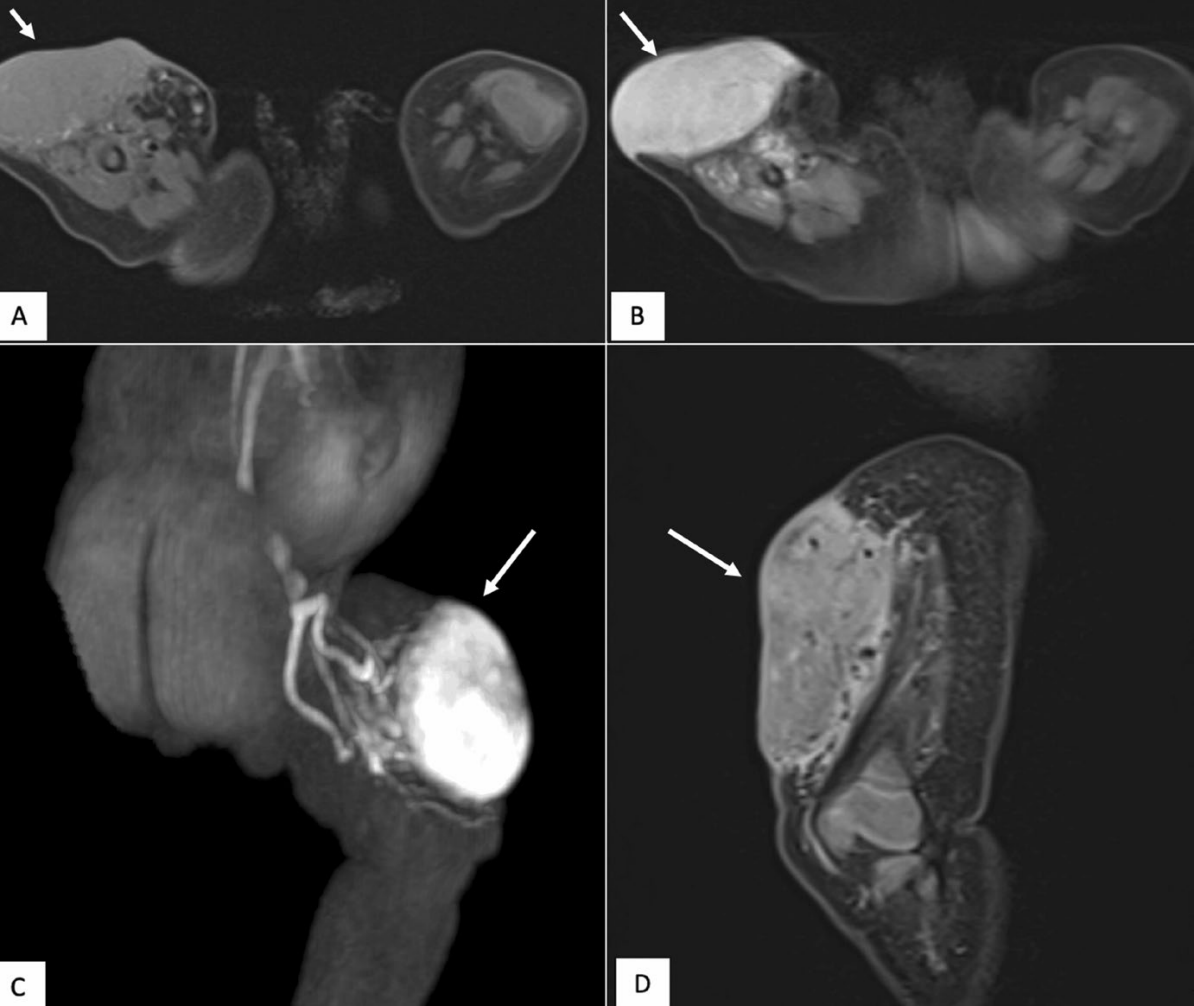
MRI Imaging of Subcutaneous Hemangioma in the Right Thigh and Knee. The white arrow indicates the lesion observed. A: Axial T1‐weighted MRI without contrast, showing a hyposignal lesion in the right thigh. B: Axial T2‐weighted fat‐saturated MRI with contrast, demonstrating enhancement of the lesion. C: Magnetic Resonance Angiography (MRA) in the sagittal plane, which shows feeding arteries. Signal void areas indicate high flow in the lesion. D: Sagittal T2‐weighted MRI with contrast, which shows hyperintensity within the lesion and its infiltration into the underlying quadriceps muscles.
